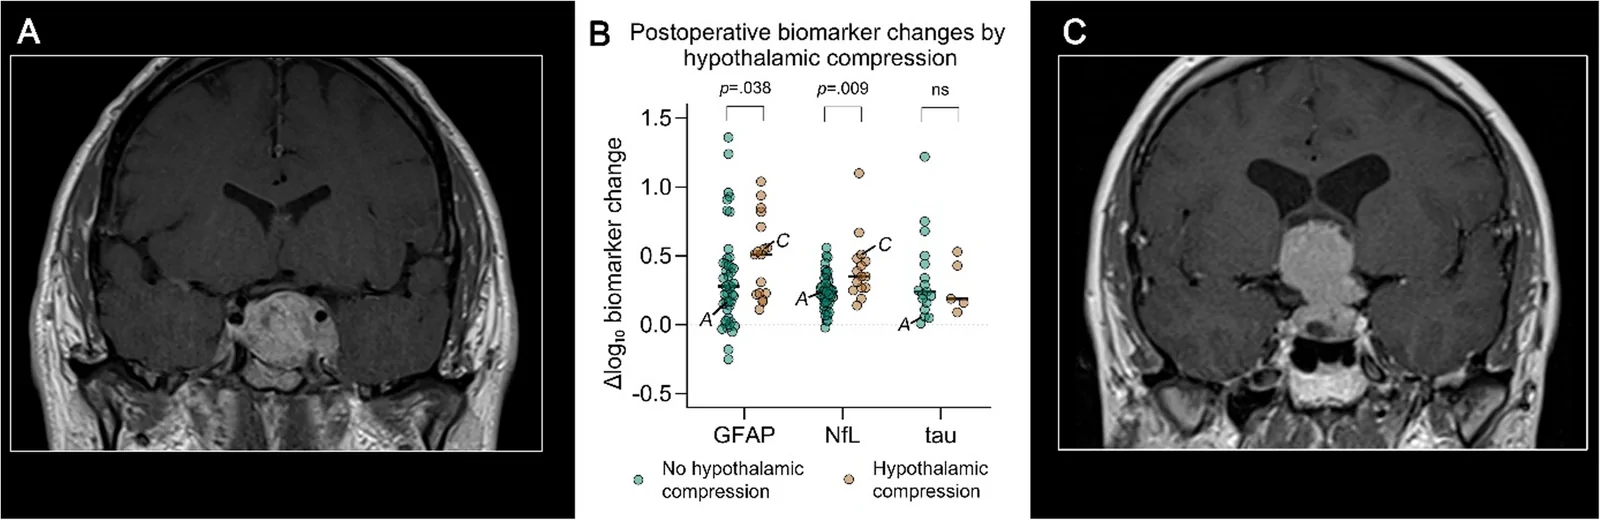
Postoperative biomarker changes related to hypothalamic compression. (A) Preoperative coronal contrast-enhanced T1-weighted MRI of a patient with a large non-functioning pituitary adenoma without hypothalamic compression. (B) Changes in plasma biomarker levels from preoperative to postoperative sampling in patients with and without hypothalamic compression. Each dot represents an individual patient. Black bars indicate medians. Statistical comparisons (Mann–Whitney U test) were performed on absolute biomarker changes, and data are plotted on a log_10_ scale for visualization. Data are from patients represented in the MRI scans in panels (A) and (C). The peak tau blood sample was unavailable for the patient shown in panel (C). (C) Preoperative coronal contrast-enhanced T1-weighted MRI of a patient with pronounced hypothalamic compression
